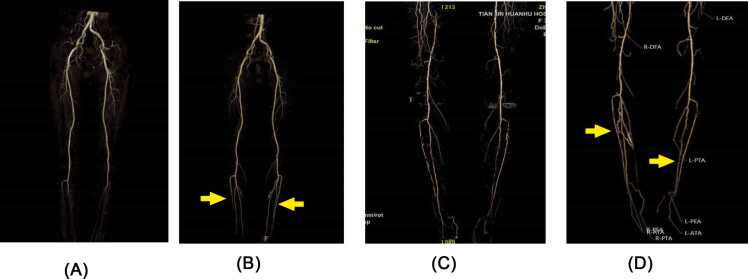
Follow-up lower extremity arterial computed tomography angiography (CTA) performed 6 months after SCS demonstrated a marked improvement in the visualization of the bilateral infrapopliteal arteries(yellow arrowhead) compared with the preoperative images. (A, C) Preoperative images; (B, D) Postoperative images at 6 months after intervention.
